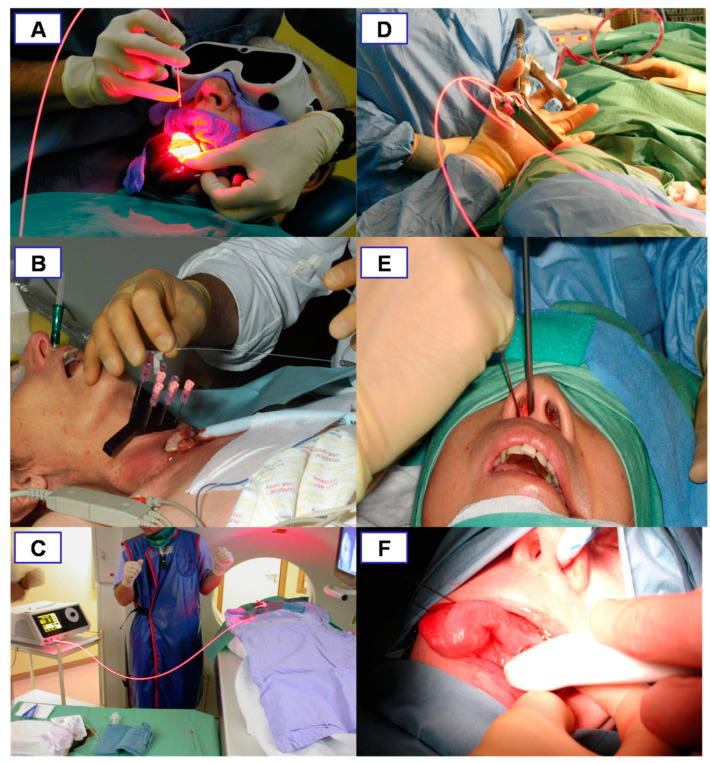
Mode of light delivery. (A): Superficial light application using a fibre-guided laser. (B): Interstitial photodynamic therapy using a pre-made grid to insert needles. (C): Interstitial photodynamic therapy with intraoperative MRI scanning. (D): Laryngoscopy employed to guide Photodynamic therapy(PDT). (E): Nasoendoscopy employed to guide PDT. (F): US employed to guide PDT. Reprinted with permission from ref. [10]. Copyright 2010 Clin. Oncol. (R. Coll. Radiol.)
